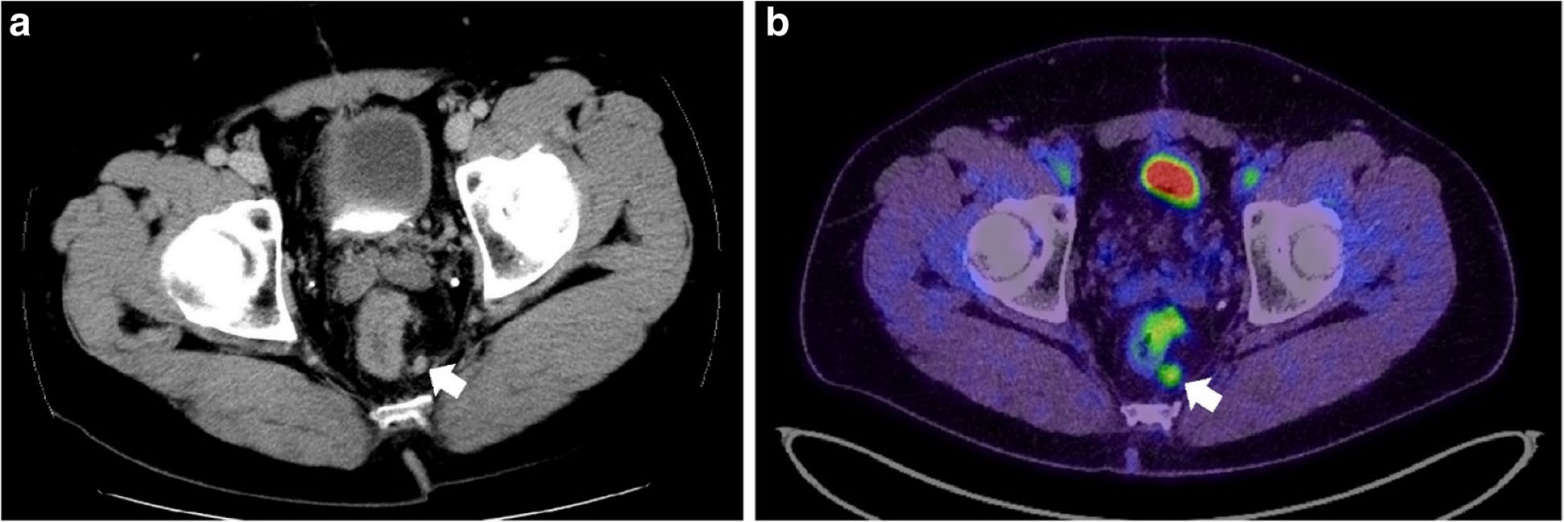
Imaging of local recurrence after secondary LAR for intramural recurrence. CT detected a small nodule which may have been lymph node recurrence in the preserved mesorectum after TSME at the bottom of the pelvis (arrow) (a). Abnormal fluorodeoxyglucose uptake was observed on PET-CT (arrow) (b)
